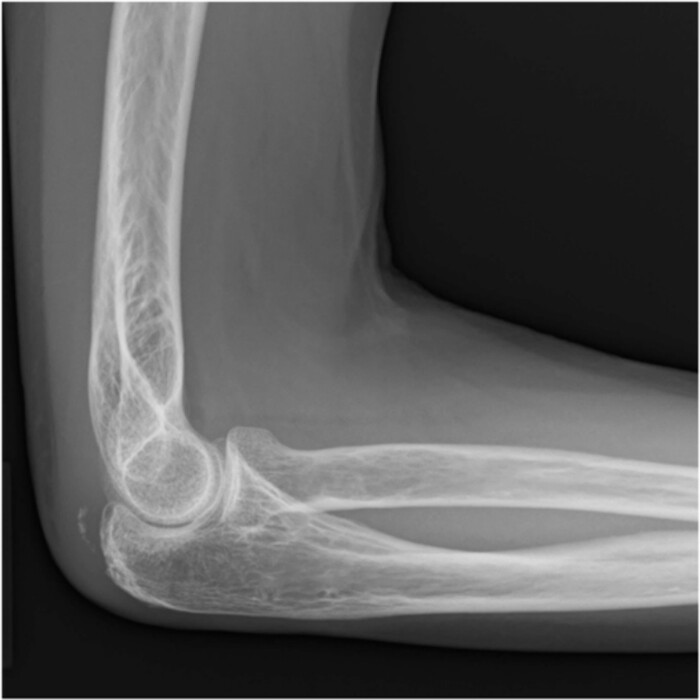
At 4 months after the surgery, the X-ray shows complete bone union in the lateral view.
